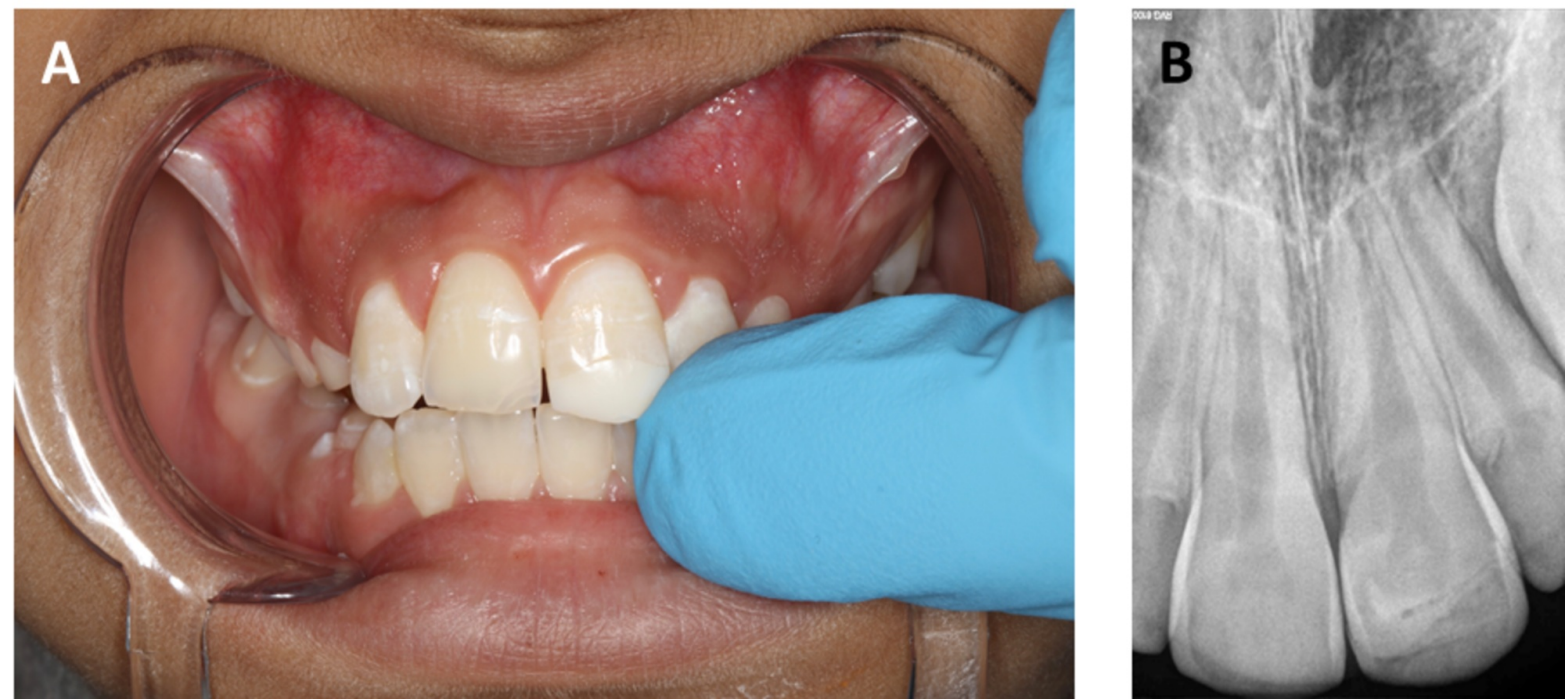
Post-operative image and radiograph. (A) Reattachment of the broken tooth fragment in the maxillary central incisor. (B) Peri-apical radiograph immediately after the attachment of the broken fragment.
